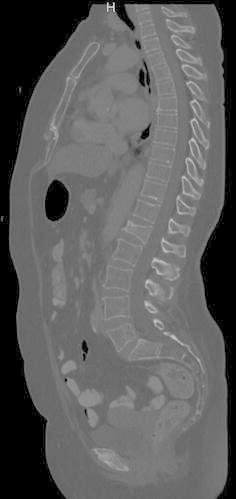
Midline sagittal reformatted CT image of a 71-year-old woman with a follow-up examination for malignant melanoma class IIIB. Vertebrae C7 to L5 were completely pictured in the computed tomography examination and were evaluated in this study. A typically appearing pathologic VBF of the first lumbar vertebrae grade 2, type wedge can be appreciated. This was reported in the impressions section of the initial radiological report; however, it was not documented in the patient’s discharge letter and, the underlying cause, be it osteoporosis or metastasis has not been determined
